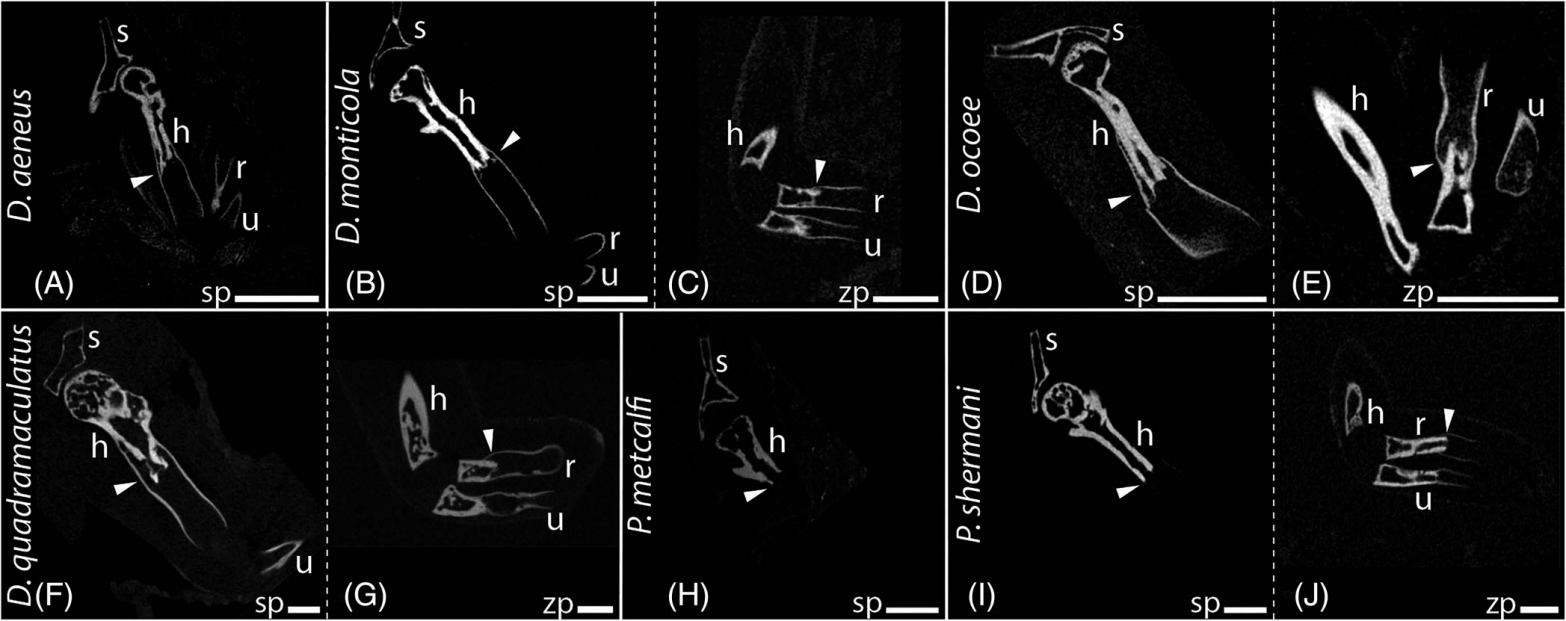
In‐lab amputations and outcomes of long‐term fore limb regeneration. Micro‐CT scans of regenerated limbs in unstained condition. *Desmognathus aeneus* (A); *D. monticola* (B‐C); *D. ocoee* (D‐E); *D. quadramaculatus* (F‐G); *Plethodon* metcalfi (H); *P. shermani* (I‐J). h, humerus; r, radius; s, scapula; u, ulna. sp, amputation site stylopod; zp, amputation site zeugopod. White arrows highlight the amputation site. White scale bars represent 1 mm.
